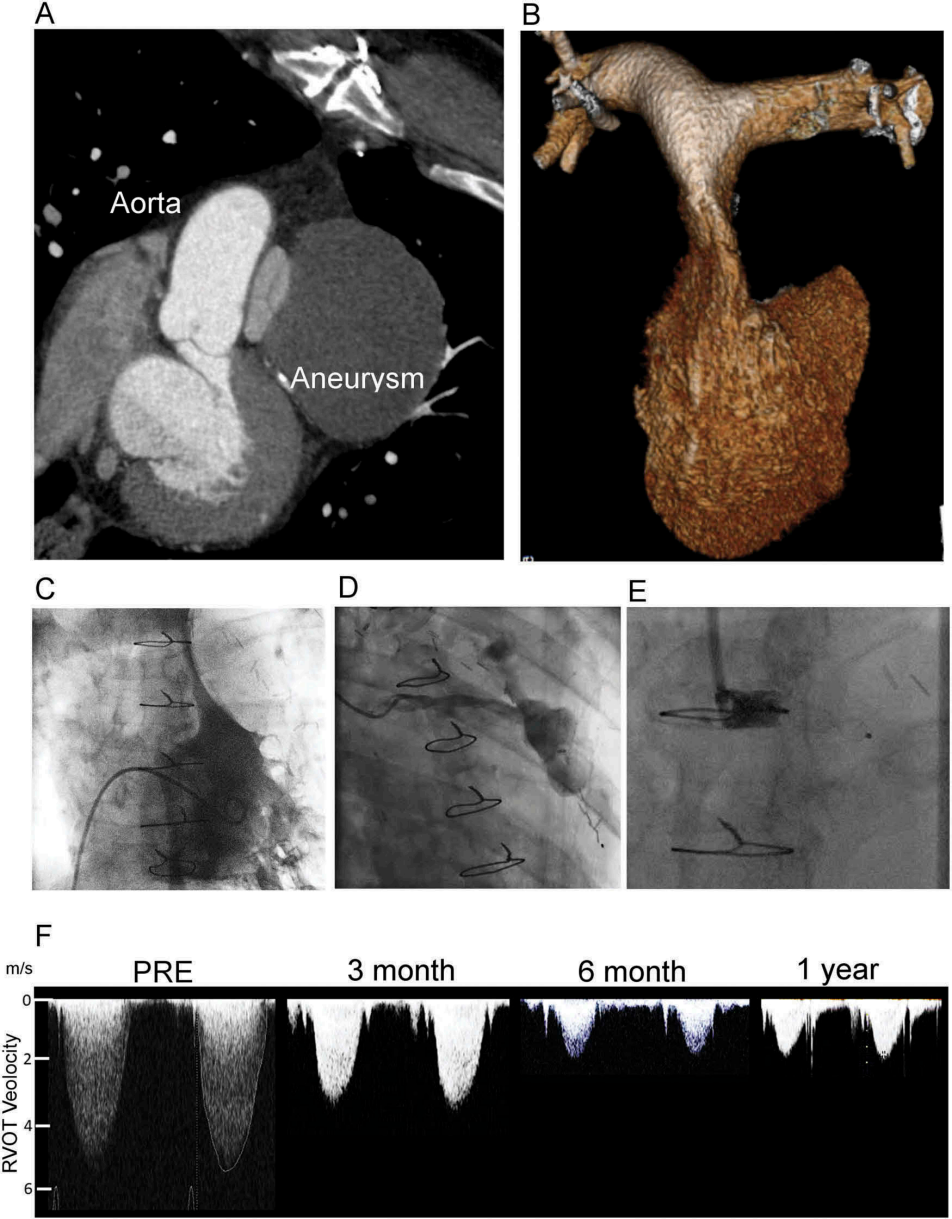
Acquired right ventricular outflow tract (RVOT) obstruction due to coronary graft aneurysm treated by percutaneous graft occlusion. (A) 2D multidetector computed tomography (MDCT) showing compression of the pulmonary valve between the graft aneurysm and the native aorta. (B) 3D MDCT reconstruction of the RV and pulmonary arteries showing the slit-like appearance of the main pulmonary artery. (C) Right ventriculography shows a minimum dimension of 2.2 mm, with an invasive pressure of 83 mmHg. (D) Graft angiography demonstrates the large aneurysm and feeding vessel. (E) An 8-mm AVPII device was used to occlude the graft with the expectation that thrombus would form and ultimately resorb. (F) Serial non-invasive Doppler assessments of the RVOT velocity show progressive and then stable reduction from 5.1 m/s at presentation to 1.7 m/s in follow up. RV dimensions, and hypertrophy normalize over this period. The patient was well and able to return to work at 6 months.
